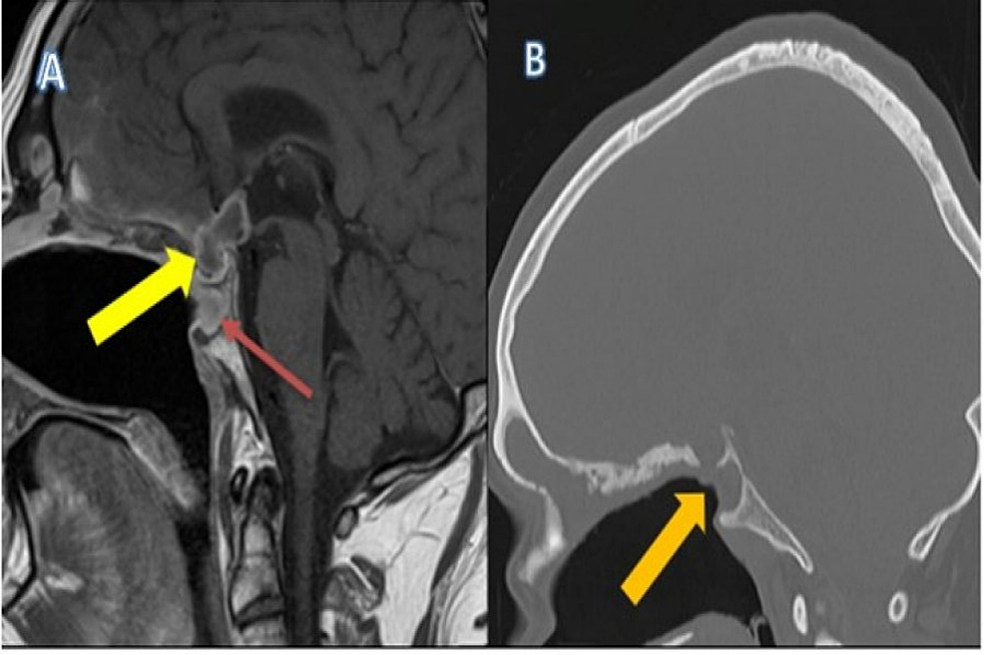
(A) MRI, sagittal T1 post contrast, demonstrating peripherally enhancing lesion arising from the pituitary fossa (yellow arrow). Enhancing soft tissue is seen in the sphenoid sinus region, adjacent to the pituitary fossa. Erosion of the Sella turcica allowing communication between the pituitary fossa and sphenoid sinus region (red arrow). (B) Right image: CT, sagittal non-contrast, demonstrating complete bony erosion of the entire septum causing loss of distinction between the paranasal sinuses (orange arrow).
